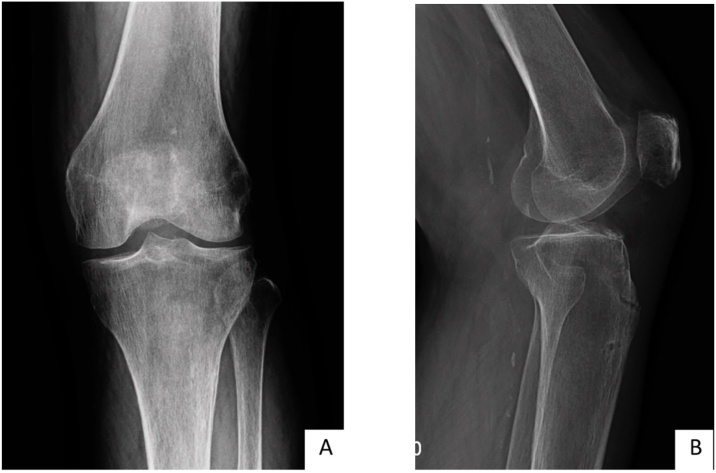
The radiographic imaging in (A) anteroposterior view, (B) lateral view of left knee at 3-months post-op.
